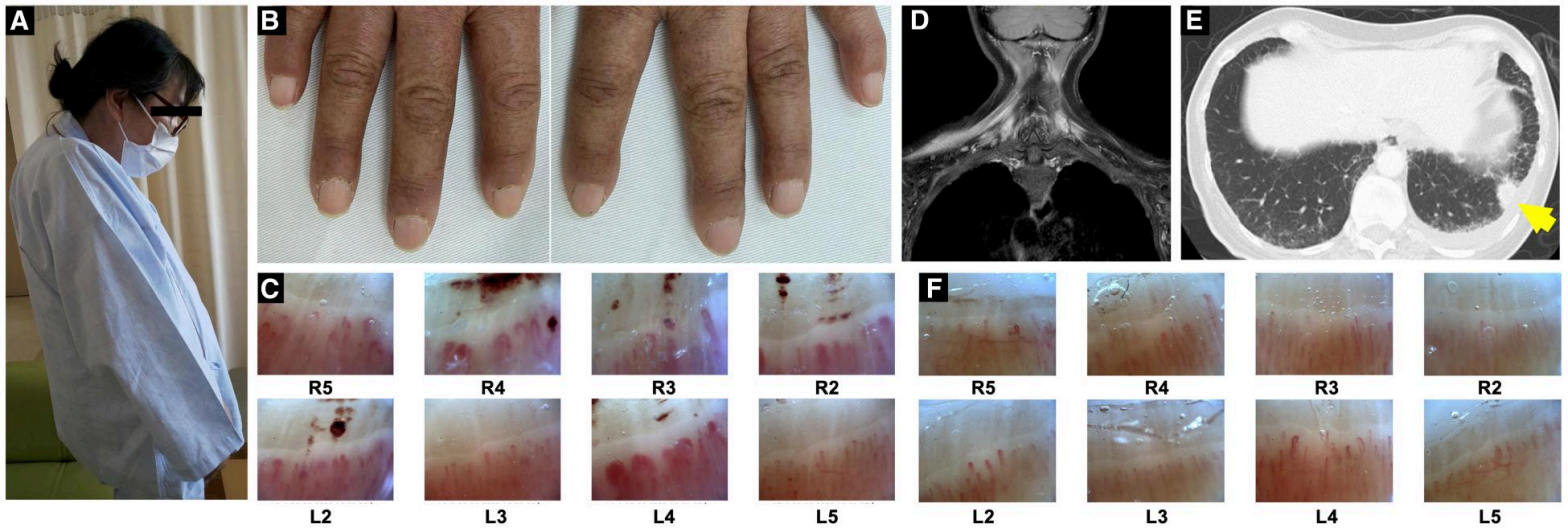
Abnormal findings seen in the patient. (A) Photograph showing the patient’s dropped head. (B) Nailfold capillary abnormalities observed in both hands. (C) Nailfold video-capillaroscopy showing dilated capillaries and haemorrhage (×200 magnification). (D) Coronal magnetic resonance imaging showing contrast enhancement in the trapezius muscle. (E) Computed tomography image showing a nodule (arrow) in the left lower lobe, along with left pleural effusion and interstitial shadowing. (F) Follow-up nailfold video-capillaroscopy performed 4 months after diagnosis showing resolution of haemorrhages and normalization of capillary morphology.
